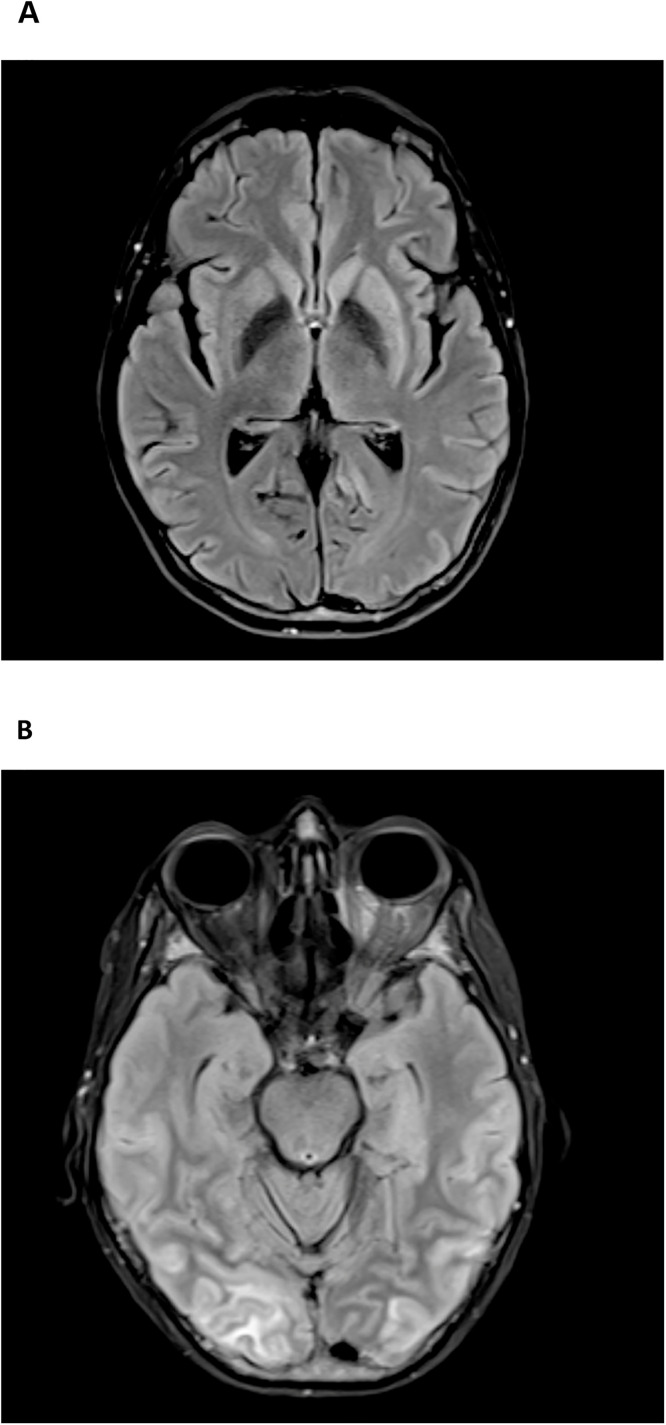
MRI findings. 2A: representative MRI slice showing T2 FLAIR hyperintense signal abnormality within the left greater than right caudate and putamen basal ganglia without evidence of superimposed enhancement. 2B: Representative MRI slice showing T2 FLAIR early signs of PRES syndrome.
